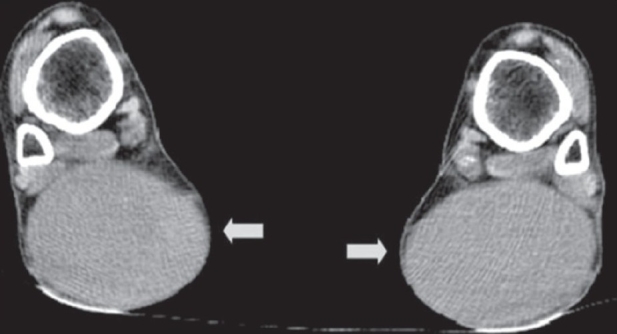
Plain computed tomography scan of both ankles reveals bilaterally symmetric soft tissue density nodules (arrows) in the Achilles tendons. No calcification, hemorrhage, or fat density is seen
